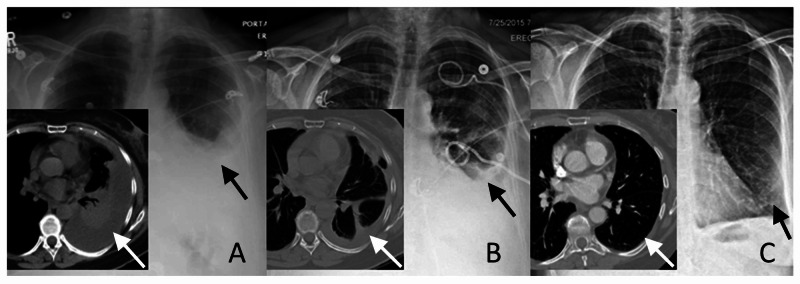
Example of Incomplete Drainage Without Surgery Representative chest x-rays with CT images inset. A 66-year-old woman presented with several weeks of dyspnea and chest pain. A) Chest x-ray suggested a moderate pleural effusion. Chest CT showed left lower lobe atelectasis and consolidation with a parapneumonic effusion. B) A pigtail catheter was placed and she was treated with three doses of tPA. Chest x-ray at completion of therapy showed persistent opacification. Chest CT demonstrated a small residual effusion with a small loculated pocket and with associated pulmonary parenchymal consolidation. The decision was made to conclude therapy and not proceed with surgery. C) Follow-up imaging at one month shows complete resolution of parenchymal and pleural abnormalities. tPA: tissue plasminogen activator.
